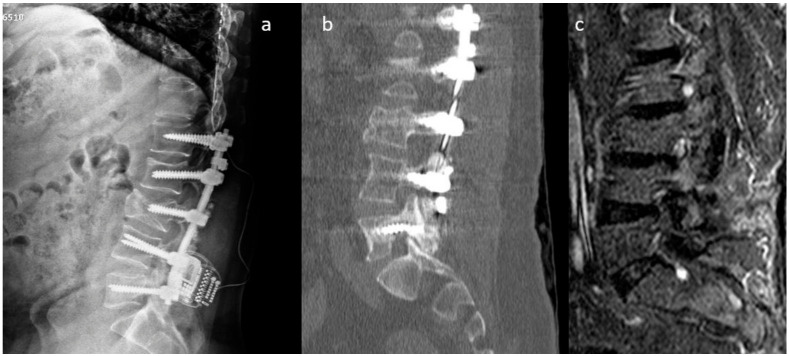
Distal clinical adjacent segment disease with implant-end compromise and severe caudal stenosis in a 58-year-old man. (a) Lateral radiograph obtained two years after surgery in a patient with worsening pain, showing long posterior instrumentation and an implanted neuromodulation pulse generator. (b) Sagittal computed tomography image demonstrating loosening around the distal instrumentation, widening of the caudal facet joint space, and reduction in disc height at the distal adjacent level. (c) Sagittal magnetic resonance image showing severe stenosis at the distal adjacent segment. This case represents a distal clinical ASD phenotype in which pain recurrence, implant-end loosening, facet distraction, disc-height loss, and severe adjacent stenosis converge into a mechanically and clinically meaningful failure pattern.
